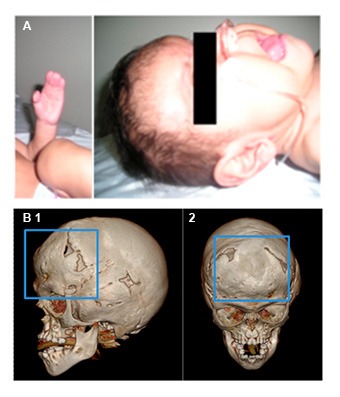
A. Male patient (II, 2) diagnosed with Apert Syndrome. Craniofacial features includes midface hypoplasia, low-set ears, depressed nose bridge, turricephaly, and osseous syndactyly in hands and feet. B. Skull computer tomography (CT) with three-dimensional (3D) model reconstruction of patient II, 2, Family 1. Several sutures synostosis (blue box) and midface hypoplasia shown in lateral (1) and frontal (2) images.
